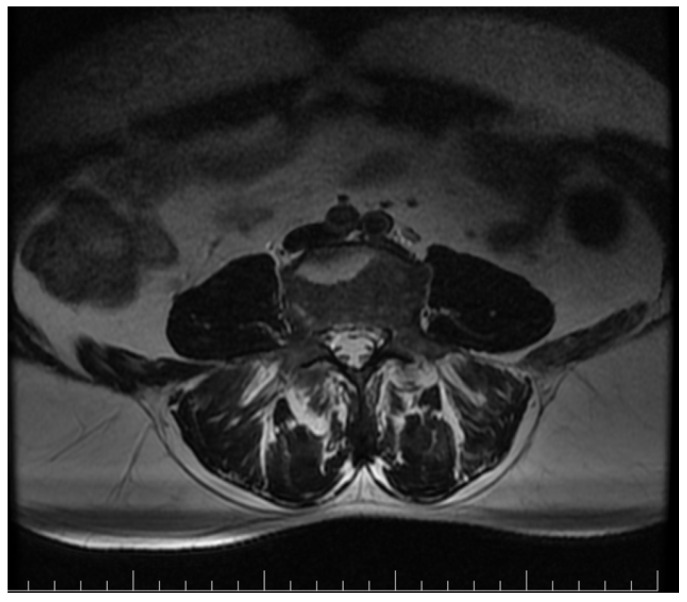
MRI showing inflammatory sacroiliac changes, including a geode-like subchondral cystic lesion and subchondral cystic change in the right acetabulum.
